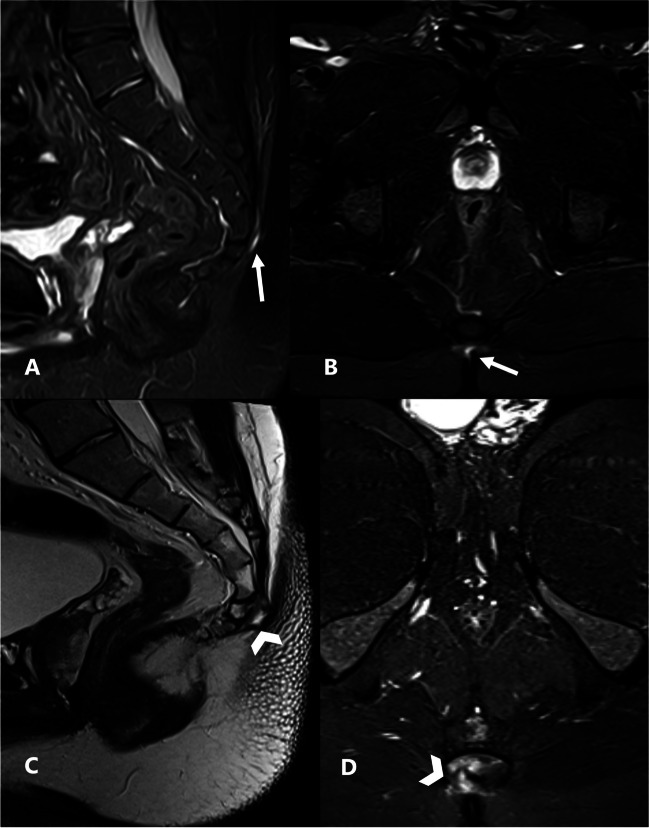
Pilonidal sinus disease in a 27-year-old male patient who underwent two consecutive MRI examinations 6 months apart with no interval surgical intervention. A and B: Sagittal and axial STIR-weighted images, respectively, of the first MRI showing a fine tract of fluid signal intensity (white arrows) seen near the posterior surface of the first coccygeal segment with normal signal of the coccyx. C and D: Sagittal T2- and axial STIR-weighted images, respectively, from the MRI performed 6 months later showing progression in size to form a small collection associated with newly developed abnormally elevated STIR signal of the adjacent coccygeal segment (arrowhead) suggestive of developing osteomyelitis of the coccyx
